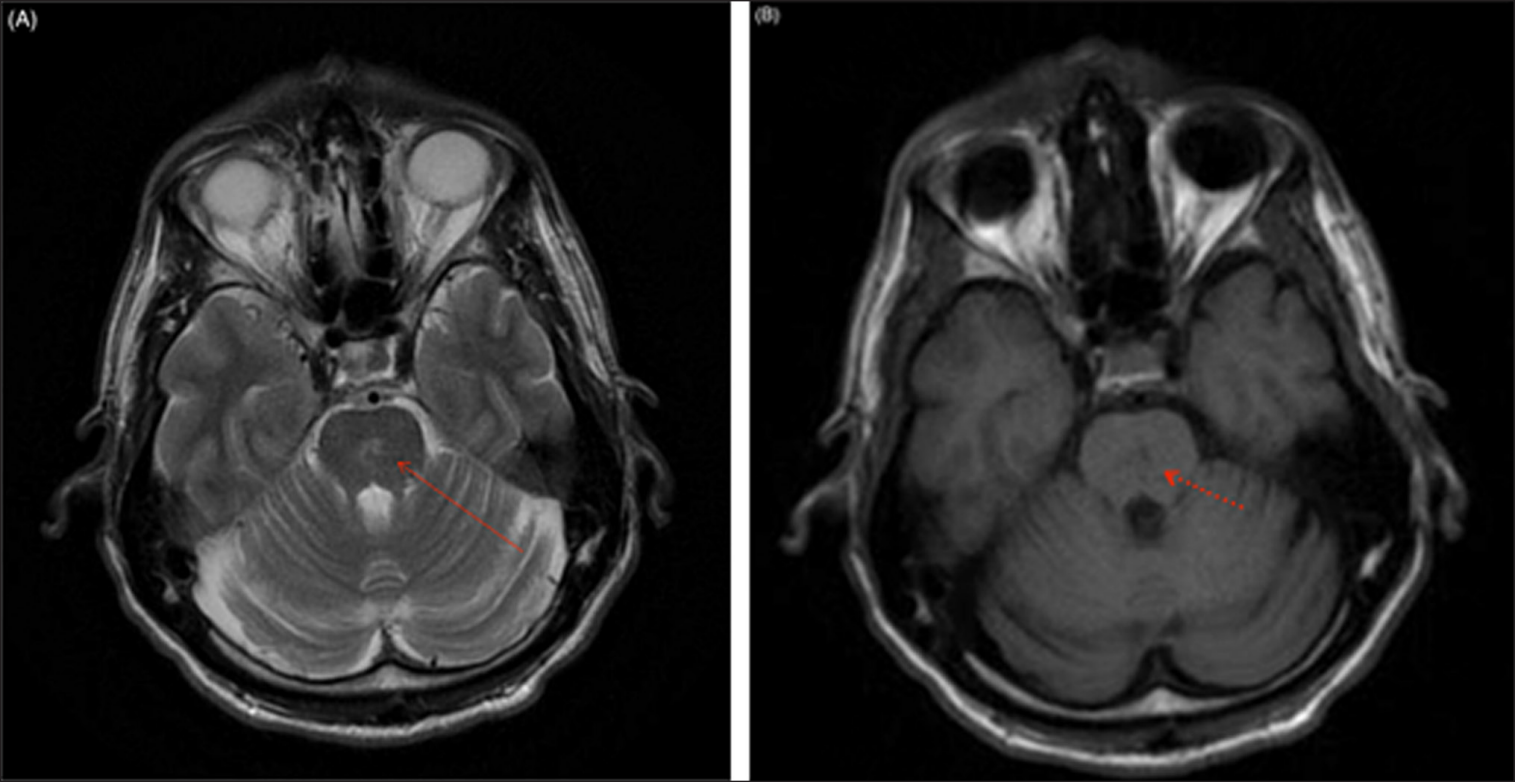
Neuroimaging in ODS. (A) Axial T2-weighted brain MRI of the patient showing hyperintensity of the central pons (solid arrow) and (B) Axial T1-weighted brain MRI of the patient showing hypointensity of the central pons (dotted arrow).
